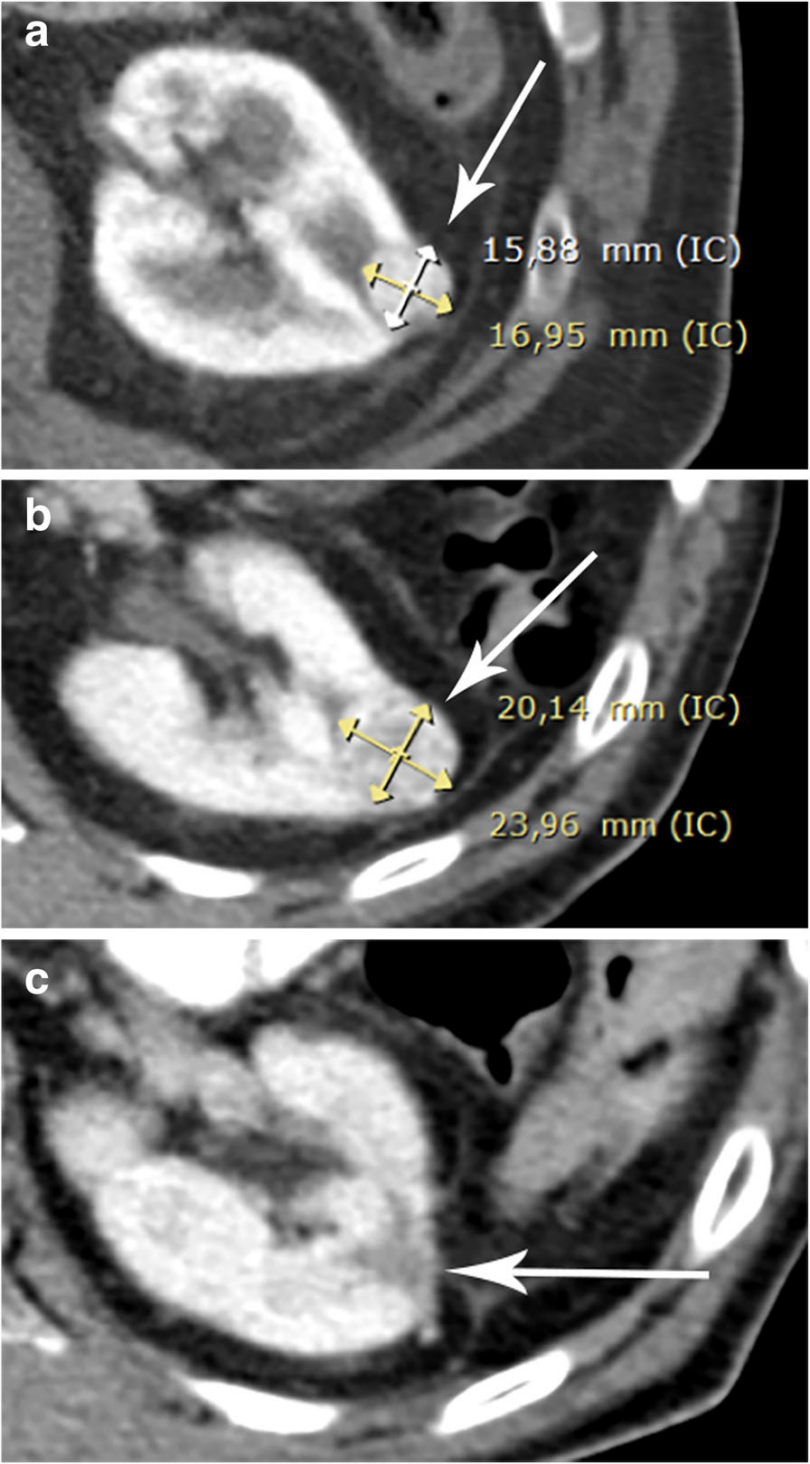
Linear GR calculation of a SRM in AS in a 73-year-old patient. a Contrast-enhanced CT of a renal mass (arrow) with a MTD of 1.7 cm (16.95 mm) at the beginning of AS and (b) 3 months later (arrow), showing a MTD of 2.4 cm (23.96 mm). Using the linear GR formula, the difference of MTD (2.4 cm − 1.7 cm = 0.7 cm) is divided by the time between the two CT scans (3 months), and the result (0.3 cm) is multiplied by 12, resulting in 2.6 cm. c Taking into account that the mass presented a GR of more than 0.5 cm/year, this mass should be considered as progressive, and AS should be discontinued. Following evaluation by a multidisciplinary committee, the patient underwent partial nephrectomy (arrow)
